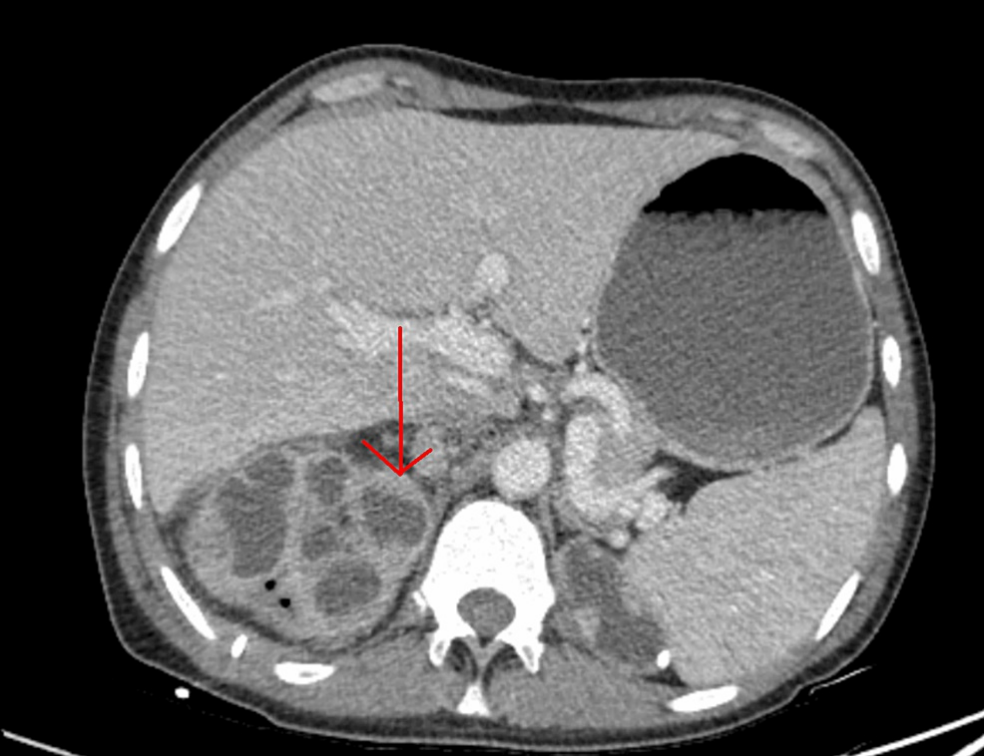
Contrast-enhanced abdominal CT scans revealed thickening of the right urothelium.
